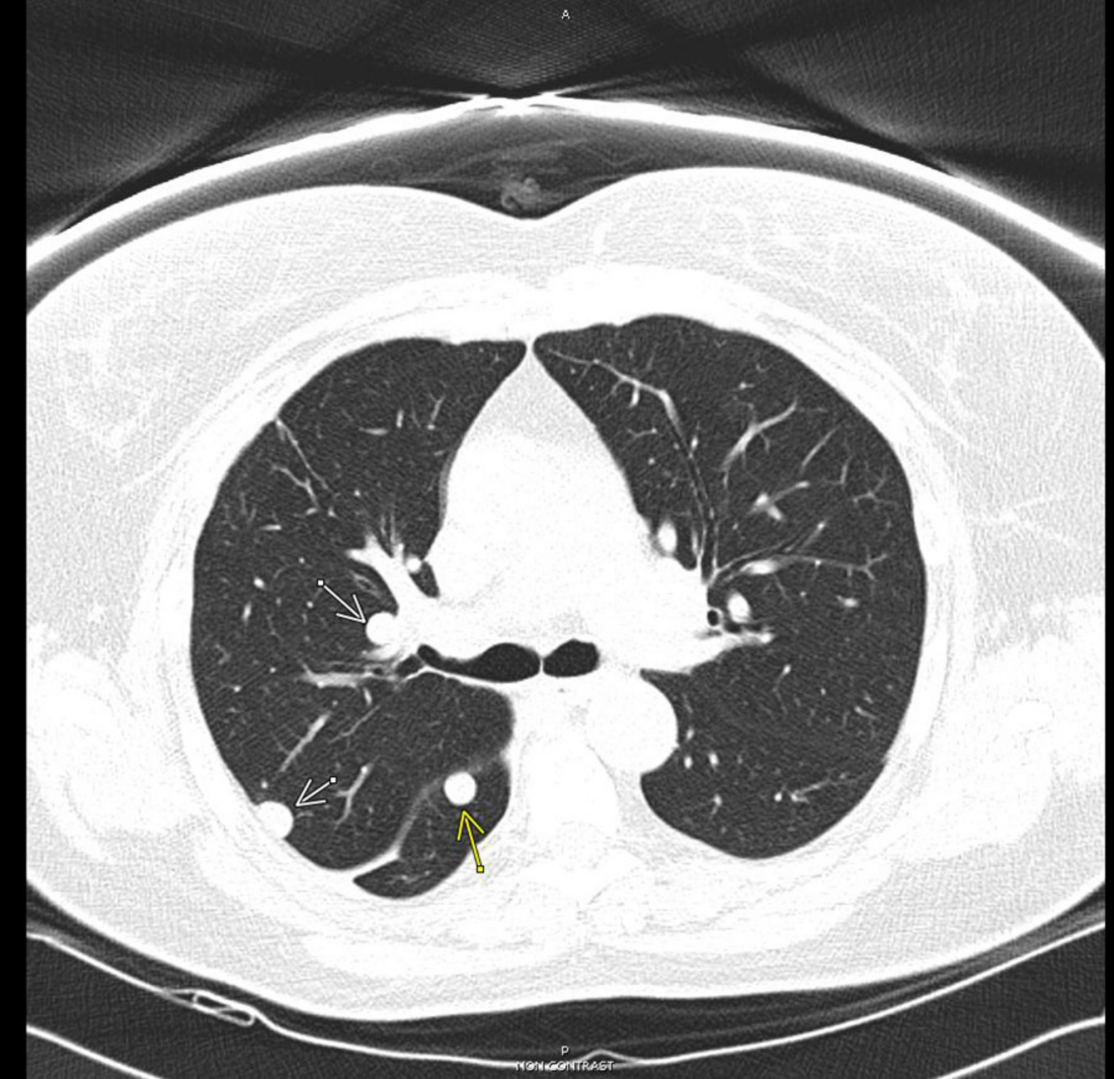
10/2016 chest CT scan showed pulmonary nodules stable in size.
